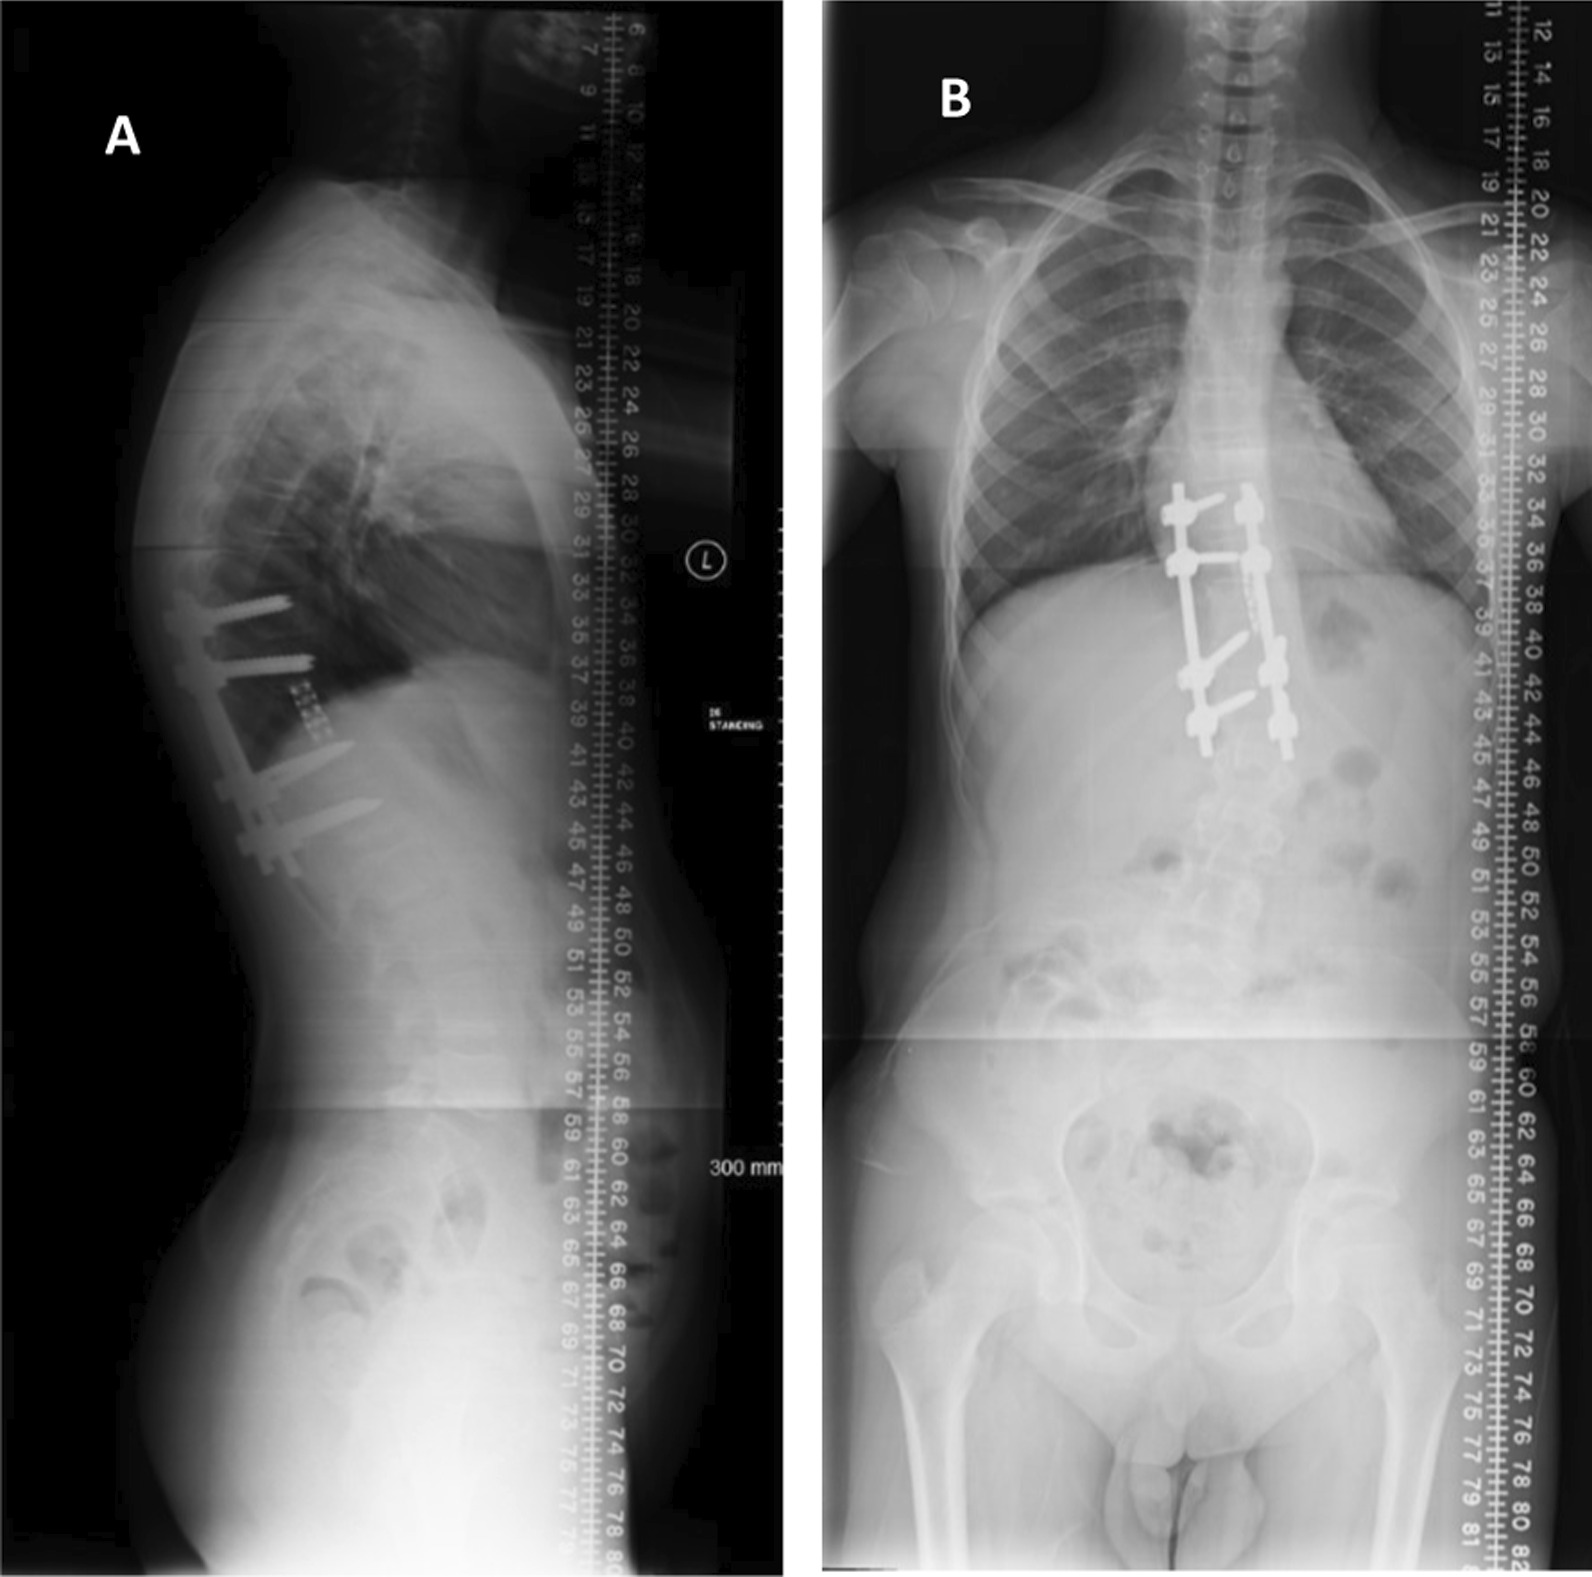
A, B Scoliosis X-ray A AP and B lateral, 1 year post first surgery showing T11 resected vertebral body with cage placement thoracolumbar fixation rods and screws with S-shape scoliosis Cobb angle of 11°
